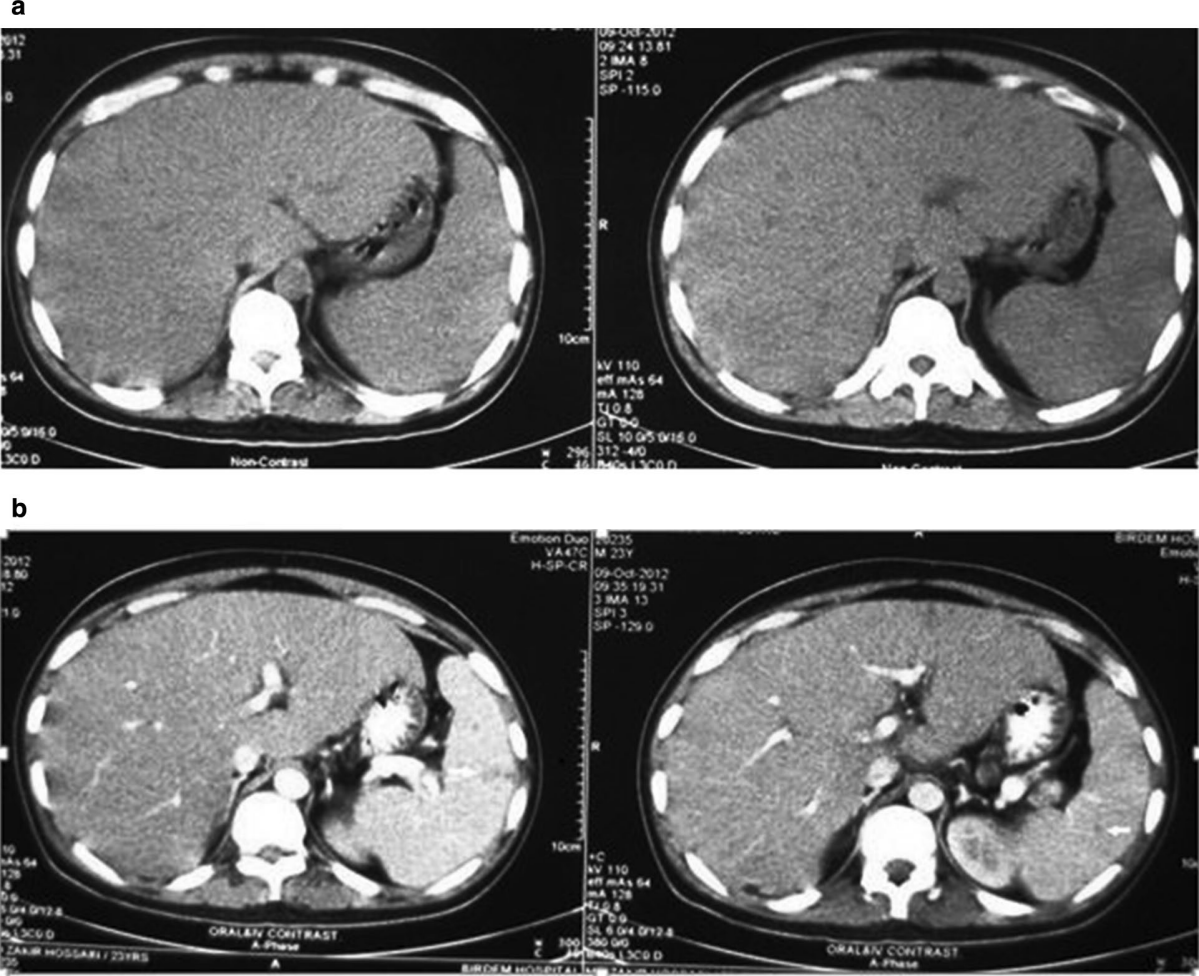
a, b Computed tomographic (CT) scan of abdomen showing hepatosplenomegaly with multiple isodense lesions in liver and spleen
